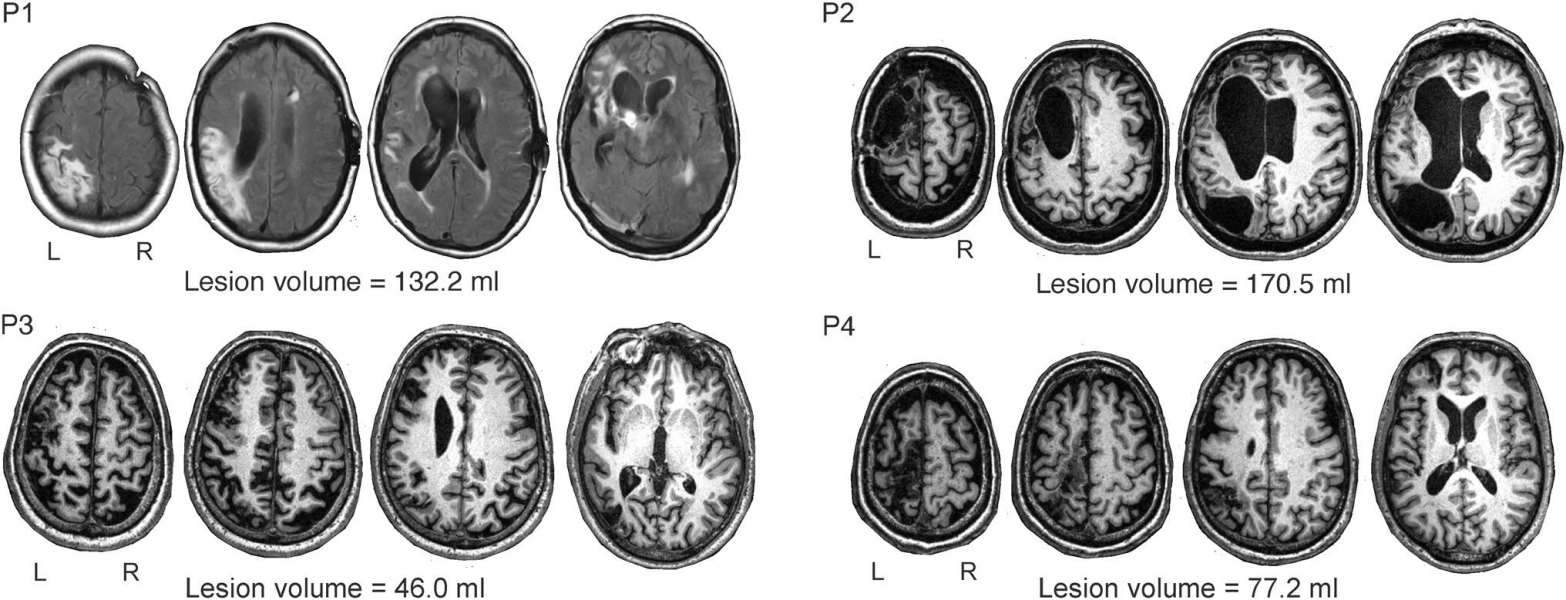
Structural MRI images showing the lesion in native space for each patient with the individual lesion volume presented below. The volume has been calculated from the image of the normalized lesion in MNI. Two white spots in P1 in the right frontal white matter substance and within the left frontal horn of the lateral ventricle correspond to the catheter for controlling hydrocephalus (further details are shown in Supplementary Results, Case Presentation). *L* left; *R* right
